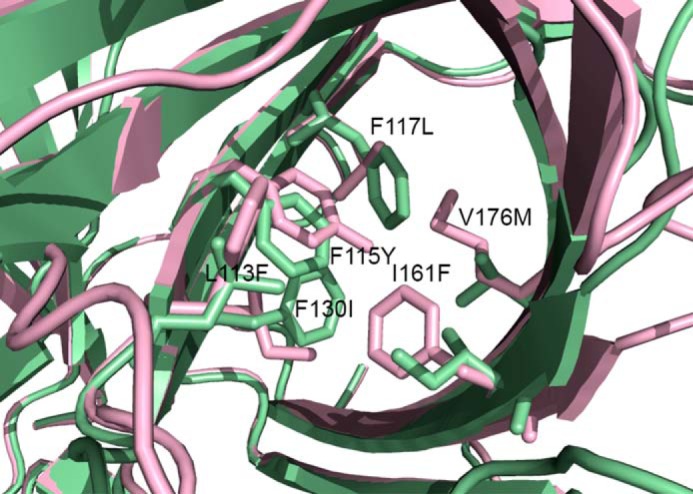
AtDIR6 homology model (*lavender*) generated from the PsDRR206 x-ray structure (*green*) on which it is superimposed. Differentially conserved active site side chains from (+)- and (−)-pinoresinol-forming DPs are shown and labeled in terms of the PsDRR206 residue type and number then the equivalent AtDIR6 residue type. (Valine 176 is substituted with threonine in the (+)-pinoresinol-forming DP ScDIR (5).)
